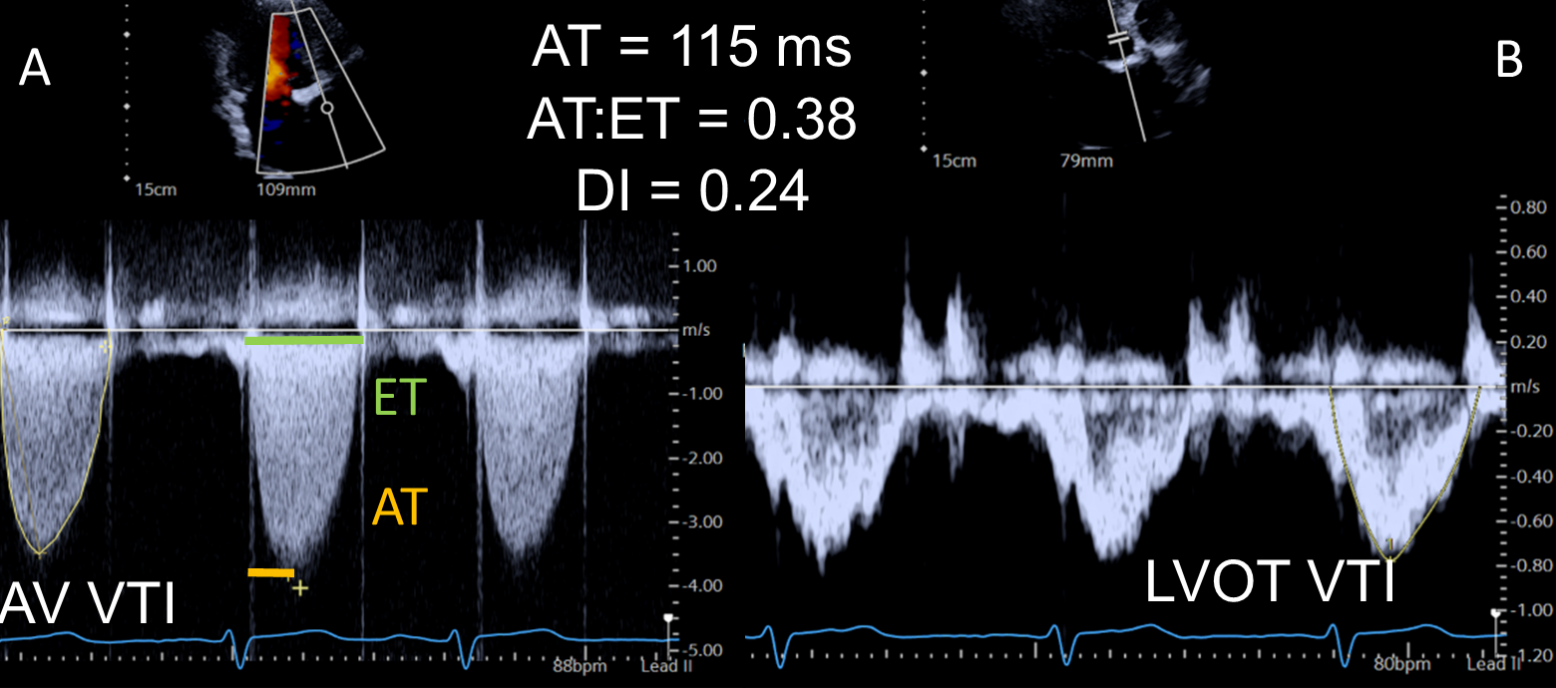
Prosthetic aortic stenosis on transthoracic echocardiography.
